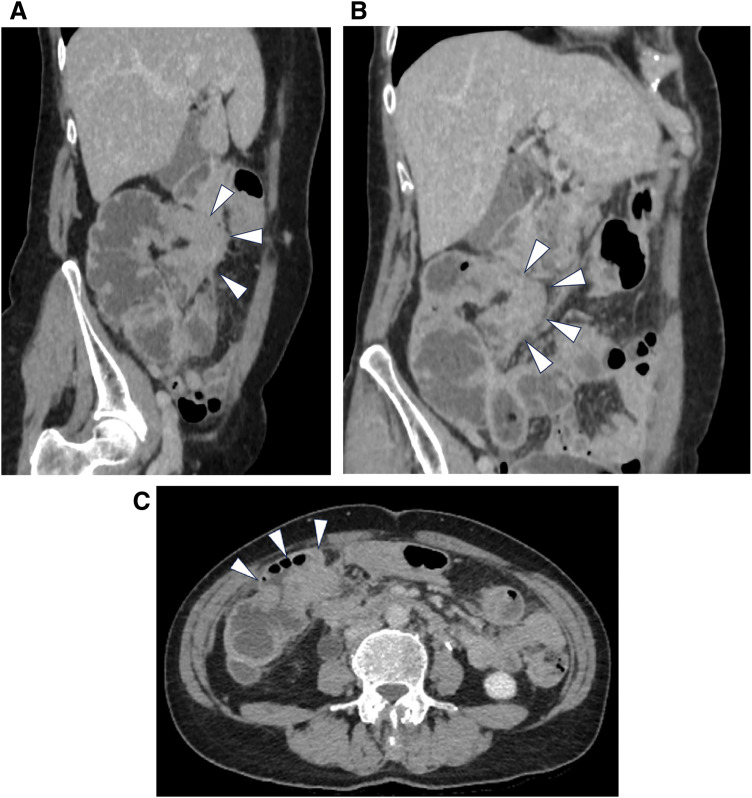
Contrast-enhanced CT of the abdomen. Wall thickening and obstruction of the transverse colon near the hepatic flexure were observed, without associated small intestinal dilation (arrowheads). No lymph node enlargement was noted. (A) Coronal section; (B) Sagittal section; (C) Axial section.
